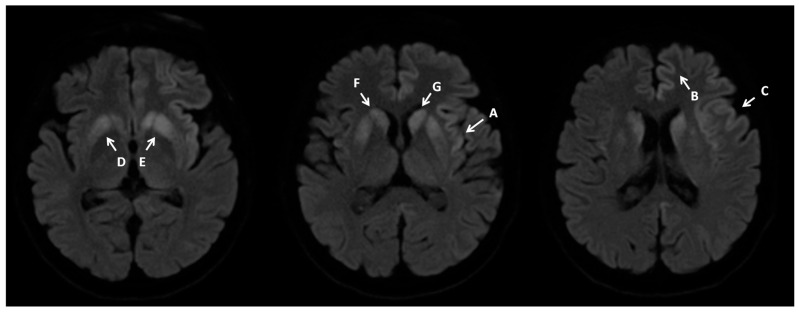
Contrast MRI imaging 12 February 2025. Imaging performed on a 3T scanner. Brain MRI shows increased signal on diffusion-weighted imaging (DWI) in the cortical gyri of the left hemisphere more intense in the area of the insular cortex (A)-cortical ribbon sign (B,C). Furthermore relatively symmetrical increased signals are visible in the lenticular nuclei (D,E) and the head of the caudate nucleus (F,G).
